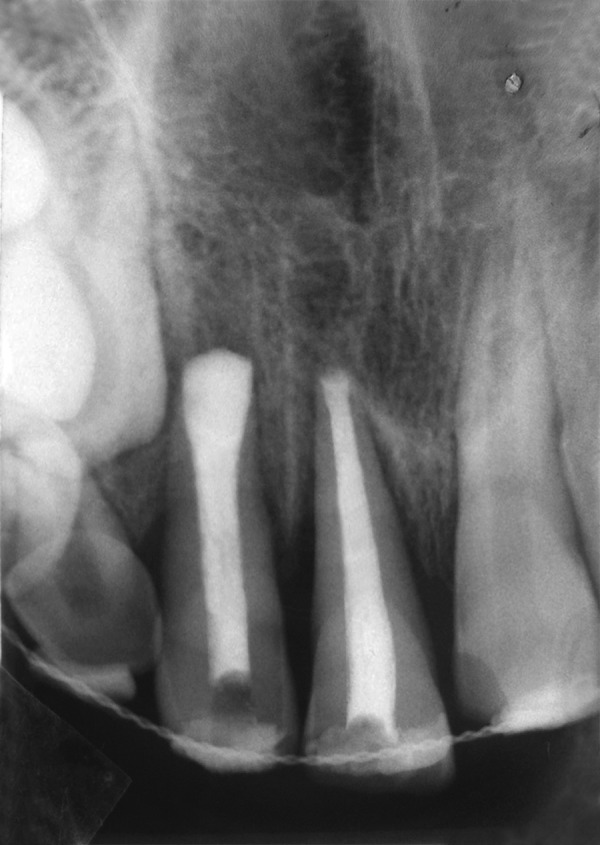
Immediate postoperative radiograph with splint in place
